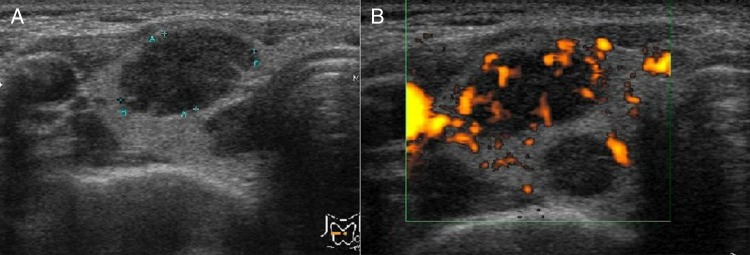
The thyroid ultrasound examination shows a tumour mass in the right lobe of the thyroid gland of ∼16 mm in diameter. Vacularisation is abundant within the mass.
